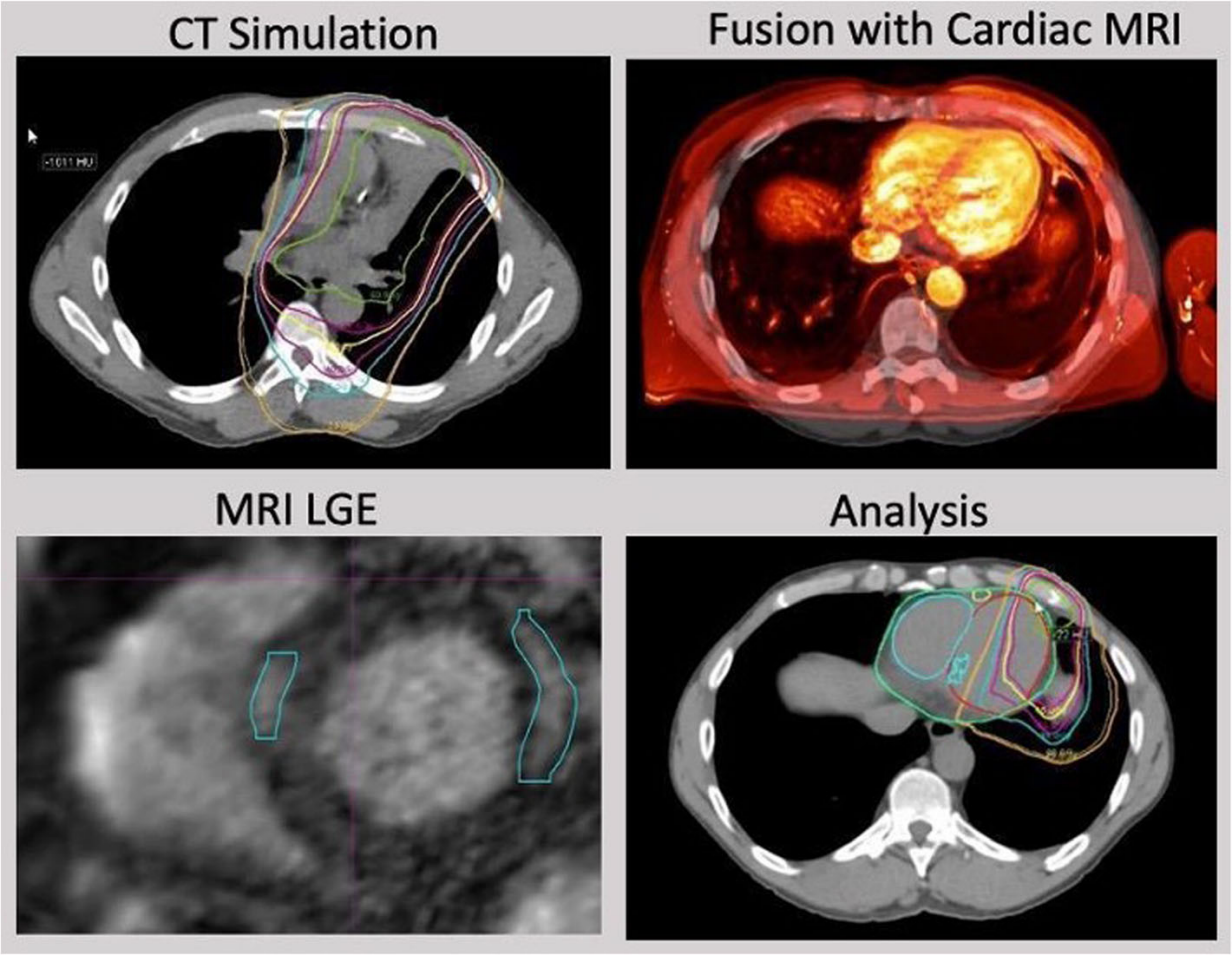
Image and dose registration process. Planning CT and associated radiotherapy treatment plan (top left) is rigidly registered with CMR (top right). Areas of LGE are delineated on CMR (bottom left). After image fusion, isodose distribution (colored lines represent areas of same dose) and LGE volume (blue volume within left ventricular septum, see arrow) are overlaid for dosimetric analysis
